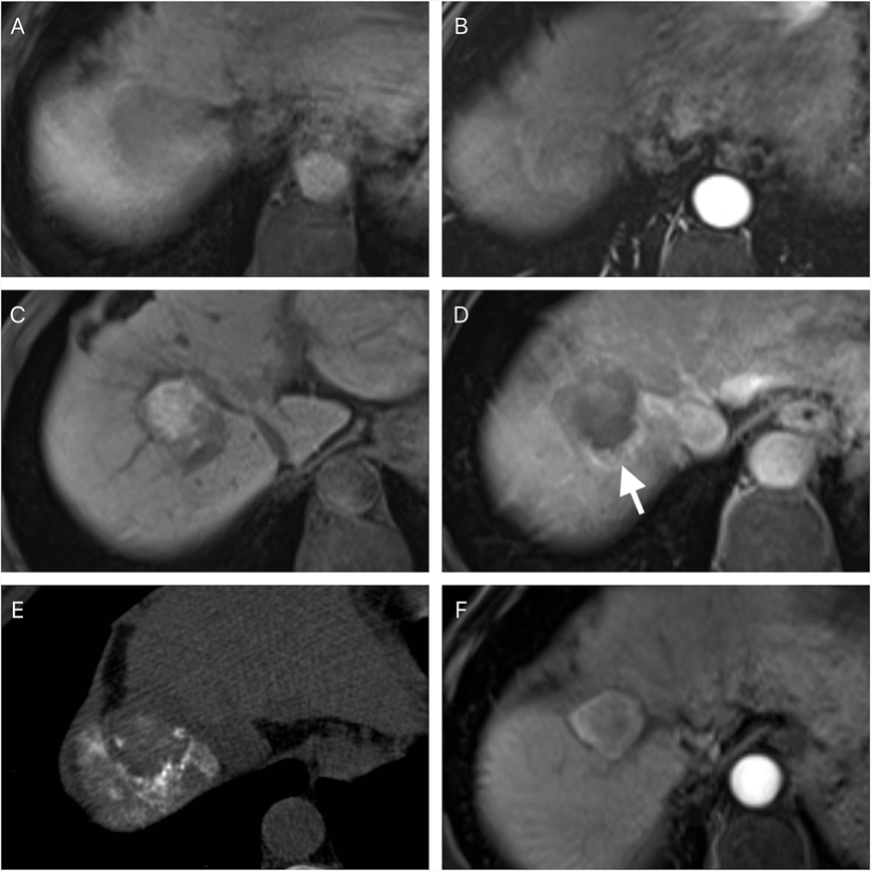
A 56-year-old male with an imaging diagnosed hepatocellular carcinoma. The pre-ablation magnetic resonance imaging showed the lesion was in the right lobe of the liver (A) and a non-rim hyperenhancement in arterial phase (B). The follow-up magnetic resonance imaging was performed four weeks after the ablation and showed residual tumor foci at the edge of the ablative zone (C, D) (white arrow). A transarterial chemoembolization was performed and iodized oil retention was noted (E). Follow-up magnetic resonance imaging was performed four weeks after the transarterial chemoembolization and showed a complete response (F).
